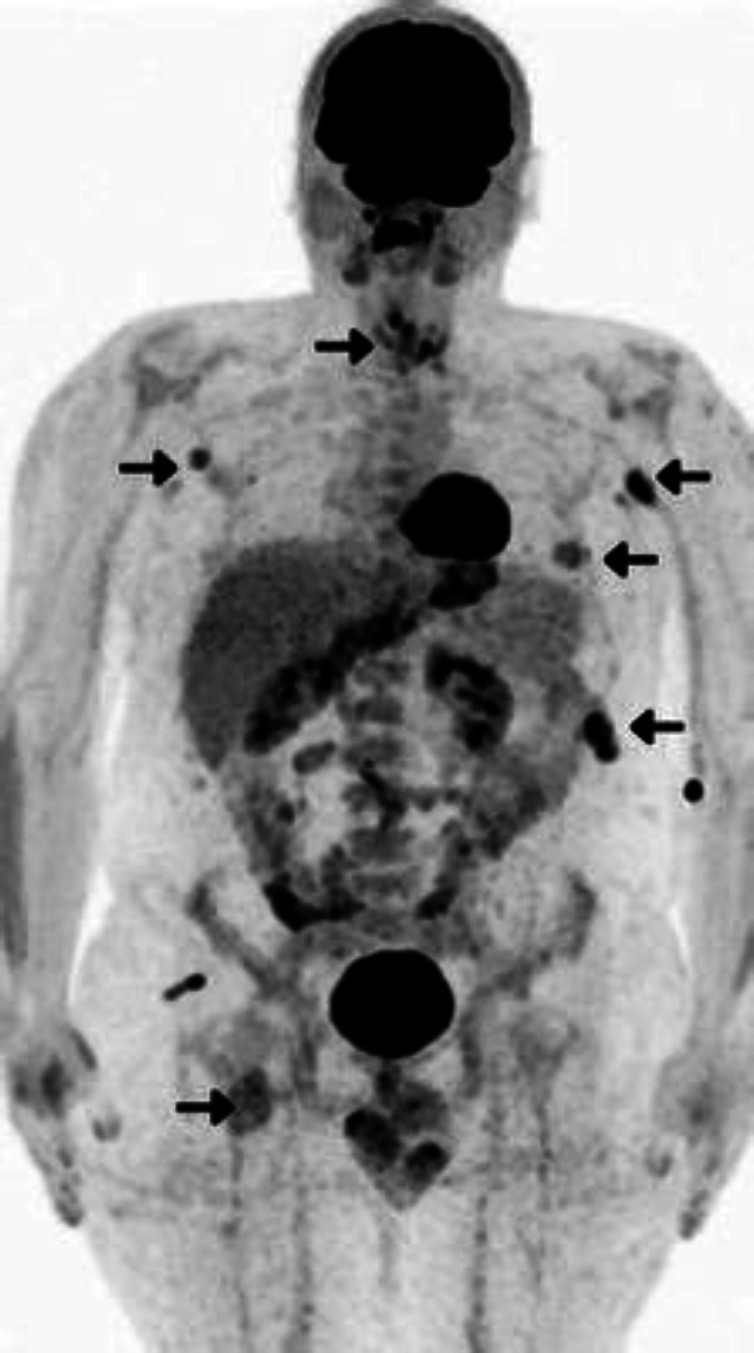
Positron emission tomography/computed tomography (^18^F-FDG PET/CT) MIP images demonstrate hypermetabolic lesions in the bilateral thyroid bed, as well as hypermetabolic two left pleural and intercostal soft tissue masses, in addition to soft tissue lesions in bilateral axillary regions and right inferior pelvis
